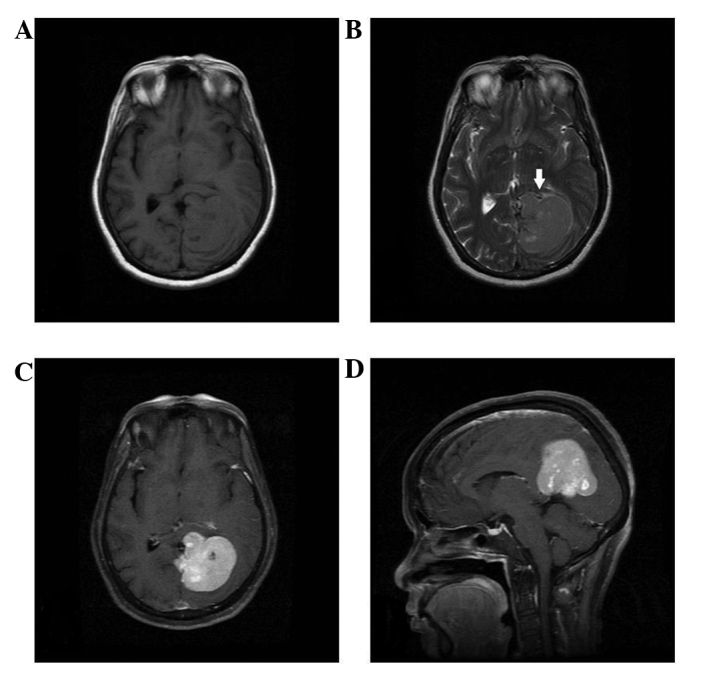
Patient 3. (A) Unenhanced magnetic resonance imaging scan reveals that the boundary of the tumor is well defined and lobular in shape. (B) Flow void can be observed in the T2-weighted image. (C and D) The lesion is markedly enhanced and the dural tail sign is not detectable.
